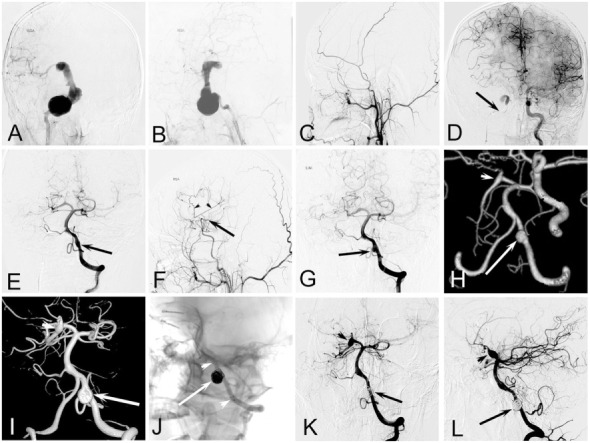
Case of an 11-year-old boy who presented with continuing headache complicated with nausea and vomiting. (A,B) Right internal carotid artery (ICA) angiogram showing a dissecting aneurysm at C4–C7. (C) Right external carotid artery angiogram after balloon occlusion of right ICA showing there was no blood supply from external carotid artery to brain. (D) Left ICA angiogram after balloon occlusion of right ICA showing the patency of the anterior communicating artery and the adequate blood supply of right anterior cerebral artery, but right middle cerebral artery (MCA) was absent. (E) Left vertebral angiogram after balloon occlusion of right ICA showing the patency of bilateral posterior communicating artery, a fusiform dilation of left vertebral artery (VA) (arrow). (F) Right external carotid artery angiogram 6 months after clipping of the aneurysm combined with superficial temporal artery–MCA bypass showing the patency of vascular anastomosis. (G) Left vertebral angiogram 6 months after operation showing the enlargement of the fusiform dilation of left VA (arrow). (H) Computed tomographic angiography 2 years after operation showing the further enlargement of the VA aneurysm (arrow) and a *de novo* fusiform aneurysm at right posterior cerebral artery (PCA) (arrow head). (I) A 3D reconstruction of a left vertebral angiogram 3 years after operation showing the further enlargement of the aneurysms at VA (arrow) and right PCA (arrow head). (J) The mask image showing the aneurysm at VA was treated by stent-assisted coiling. (K,L) Left vertebral angiogram 8 months after the intervention shows perfect reconstruction of the dissecting artery, and the aneurysm at right PCA was relatively stable.
